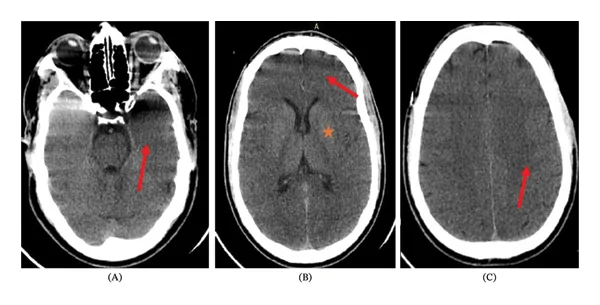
(A–C) Multilevel axial plane CT scan of the brain showing grossly hypodense brain parenchyma (cerebrum and cerebellum), predominantly hypodense subcortical regions with poor gray‐white differentiation (red arrow), and slight preservation of the basal ganglia (caudate nucleus, thalami, and lentiform nucleus [orange star]).
